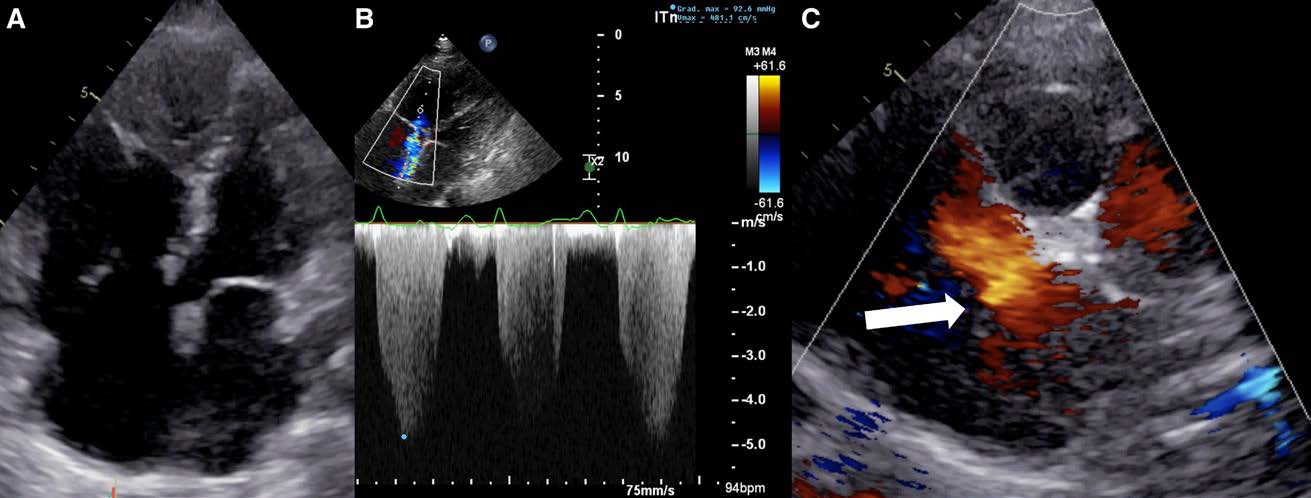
Transthoracic echocardiographic findings at presentation in the tertiary center. A, Transthoracic echocardiography at presentation showing right cavities dilatation and septal bulging into the left ventricle in apical 4-chamber view. B, Mild tricuspid regurgitation with peak tricuspid regurgitation velocity at 4.81 m/s (93-mm Hg gradient between the right ventricle and the right atrium according to the Bernoulli equation). C, Subcostal view of the so far unknown atrial left-to-right shunting (white arrow).
